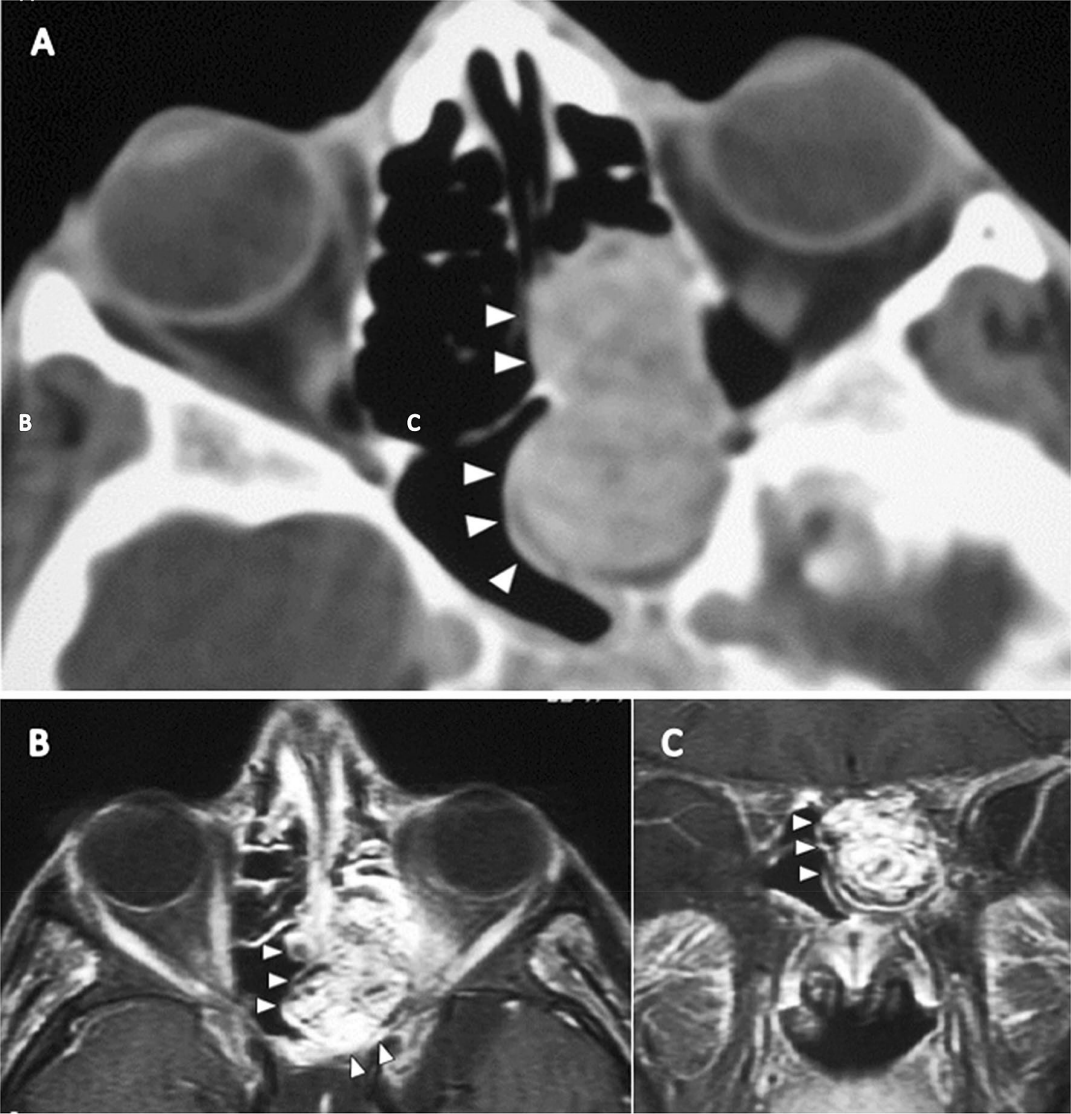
22-year-old man with a rhinopharyngeal vault PMT who presented with a 4-year history of generalized pain and progressive weakness confining him to a wheelchair. (A) Axial CT shows an enhancing bulging tumor arising from the left ethmoidal sinus with an extraosseous soft tissue component. Axial (B) and coronal (C) axial T2 weight spin-echo MR image show a lesion of the left ethmoidal sinus demonstrating an increased signal intensity of the solid mass, with multiple dark foci or vascular flow voids
